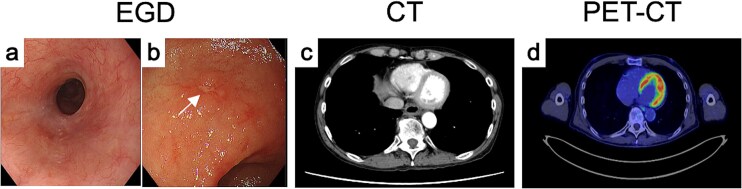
Endoscopic, CT, and FDG-PET findings after treatment. After induction chemoimmunotherapy, both (a) the primary tumor and the (b) IM markedly shrank. (c) CT and (d) FDG-PET findings of primary tumor after treatment.
